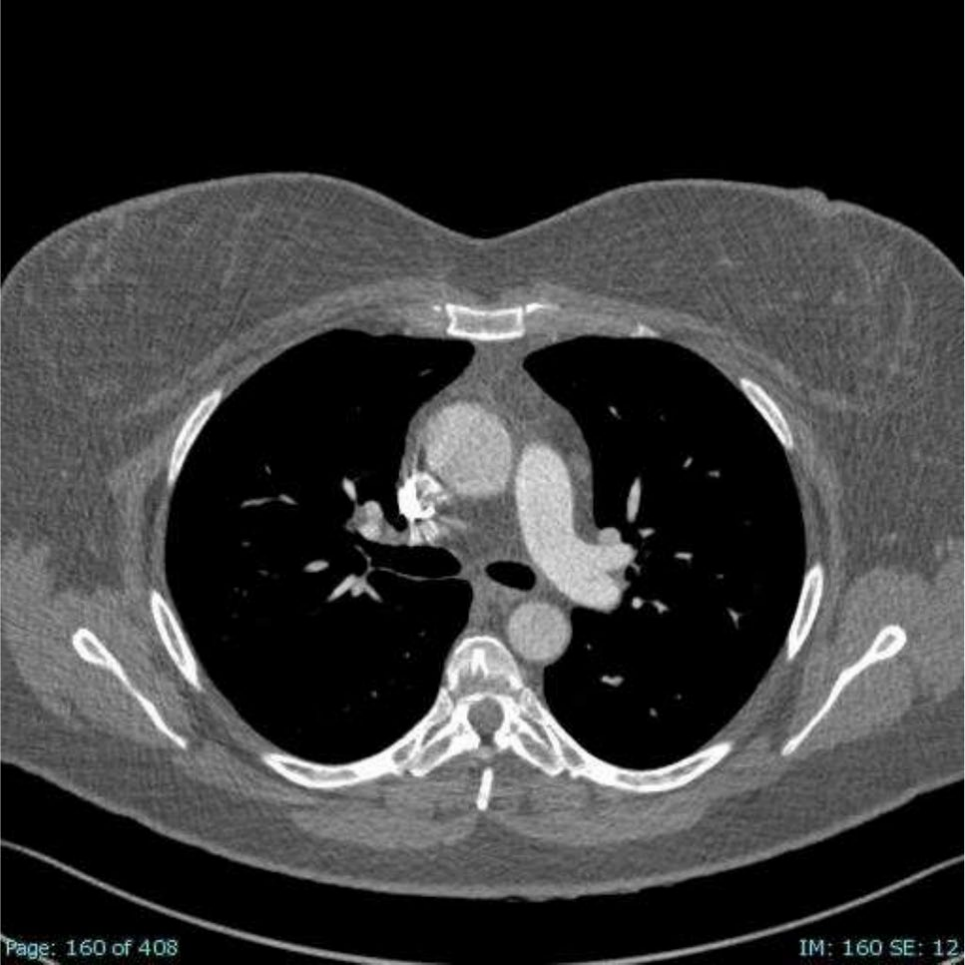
Axial CTA scan reveals submassive PE of a right subsegmental lobar artery
